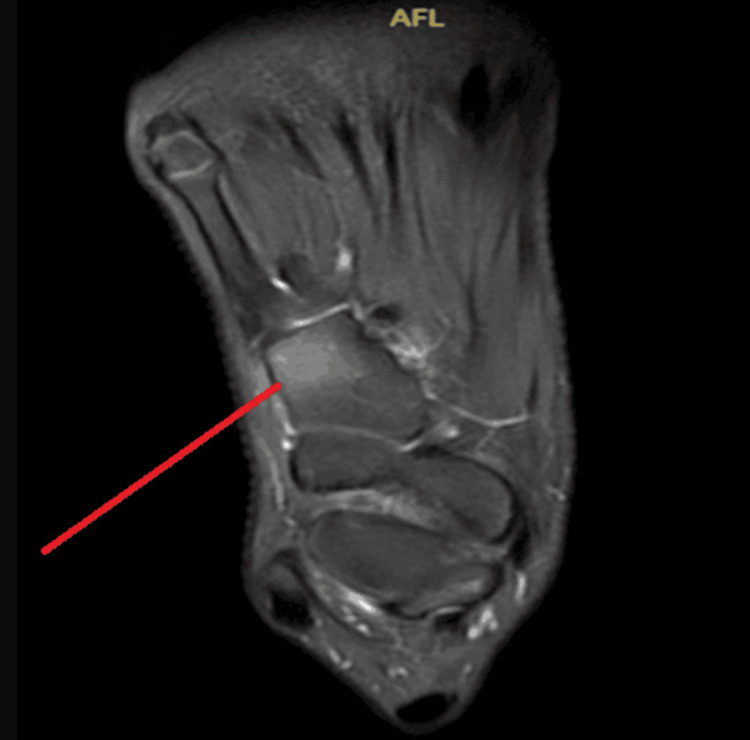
T2-Weighted Coronal MRI of the Right Foot T2-weighted coronal MRI showing bone marrow edema in the right cuboid and navicular bones.
